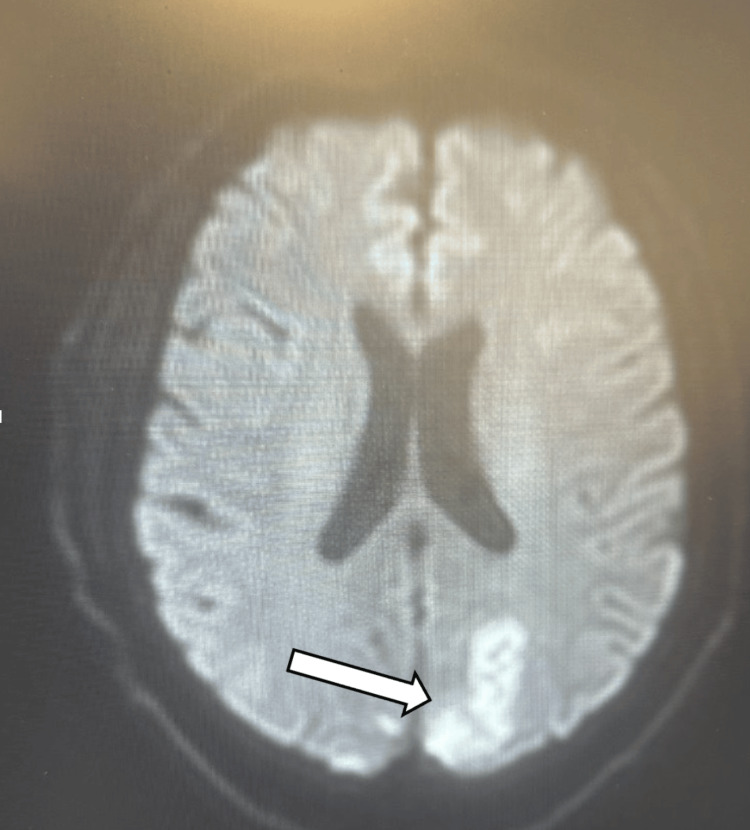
MRI brain demonstrating acute posterior circulation infarction. Axial diffusion-weighted imaging (DWI) demonstrates a focal hyperintense signal within the cerebellum (arrow), consistent with restricted diffusion and acute ischemia. These findings correlate with the patient’s presenting neurologic deficits and confirm posterior circulation infarction.
